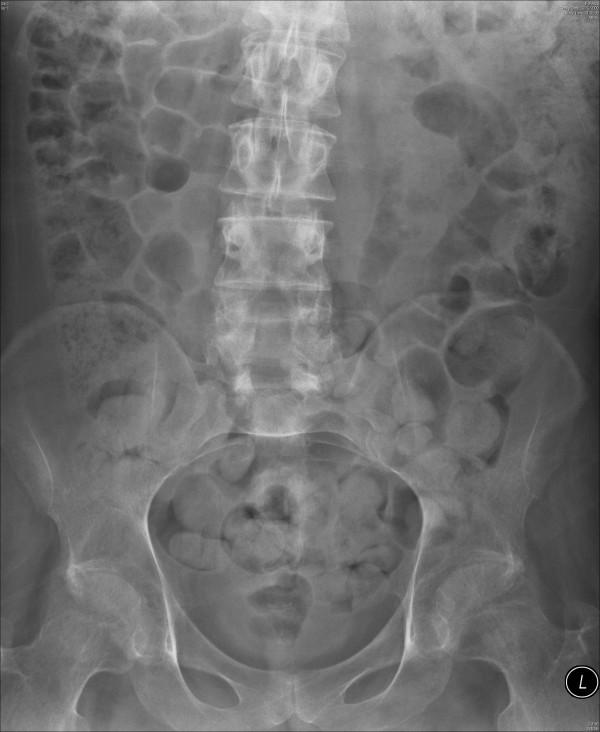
X-ray of abdomen, taken on 26 October 2009, showed marked faecal loading through the colon. Electrodes of Brindley stimulator, originally placed in sacral region, were no longer visible, as they had been removed. Please compare with Figure 1.
